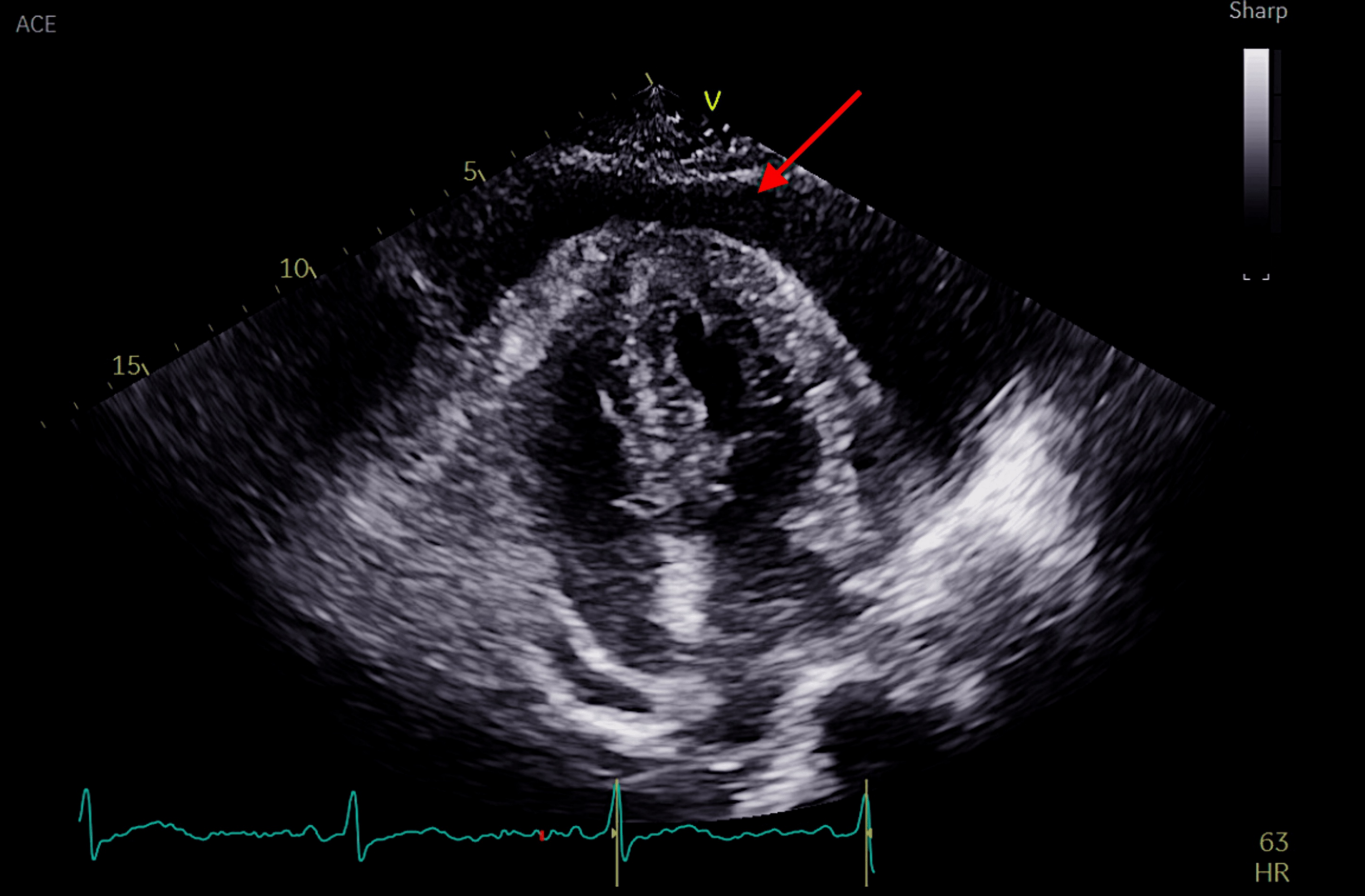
Apical four-chamber view on TTE depicting pericardial effusion (red arrow) TTE: transthoracic echocardiogram
